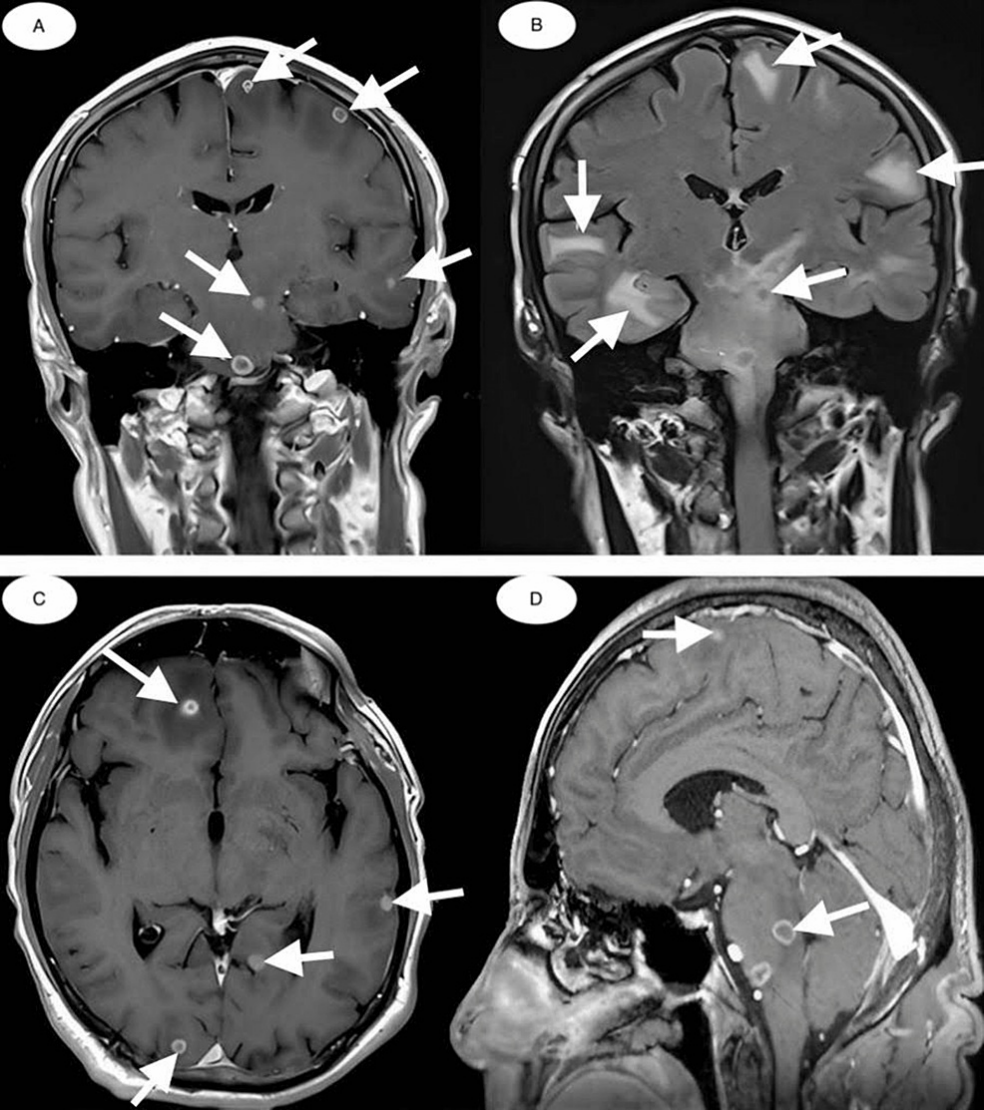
Brain CT scans in different planes (coronal, axial, and sagittal) Multiple iodophile neoplastic lesions (A, C, and D) are identified with centimetric diameters. They are located diffusely throughout the brain mass and accompanied by perilesional edema (B) in the cerebral trunk (secondary determinations).
